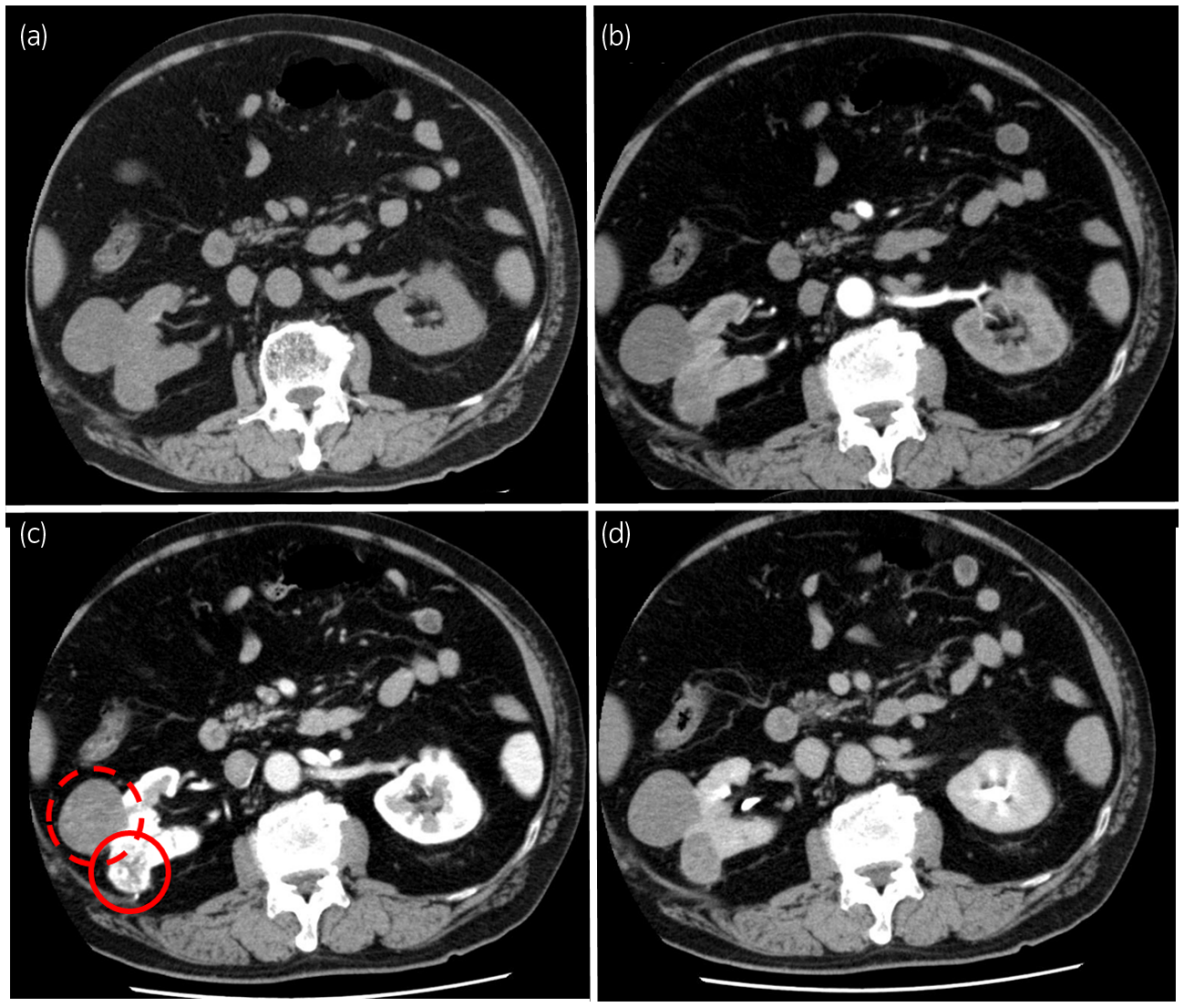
CT enhancement patterns of the two ipsilateral renal tumors in this patient. (a) Plain CT, (b) early arterial phase, (c) parenchymal phase, and (d) delayed phase. The cT1a renal tumor (red circle) showed hypervascularity on the parenchymal phase, whereas the cT1b renal tumor (dotted red circle) showed low vascularity indicated by scarce contrast enhancement.
